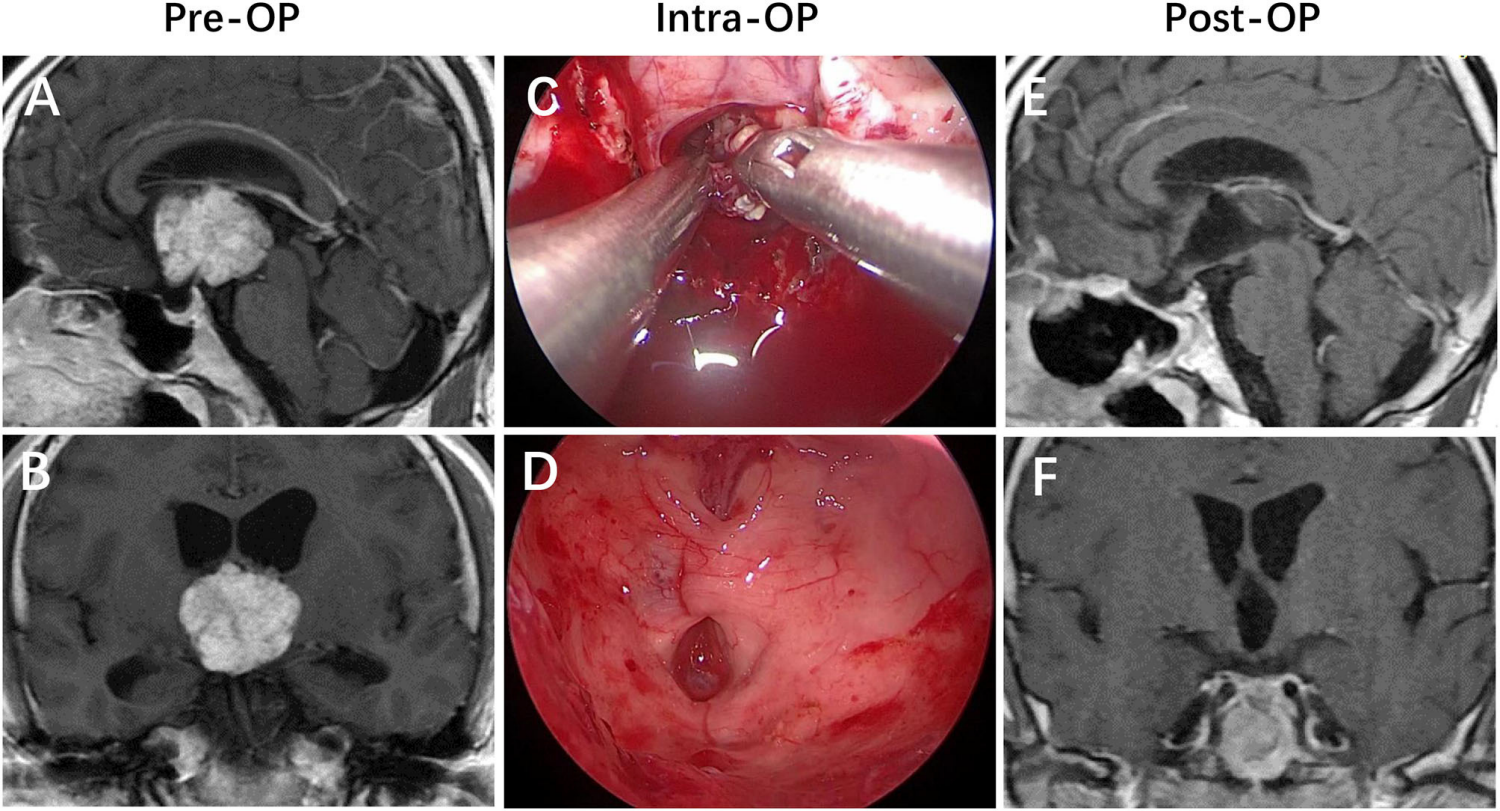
Strictly intraventricular craniopharyngioma. (A,B) Preoperative enhanced MRI demonstrated a mass that is localized in the third ventricle cavity with an intact floor lying below its inferior surface. (C,D) Intraoperative findings revealed that the patient achieved a gross-total resection of the lesion *via* the endoscopic endonasal suprachiasmatic trans-lamina terminalis corridor. (E,F) Postoperative enhanced MRI revealed that the lesion was totally removed, with the posterior wall of the third ventricle preserved intactly.
